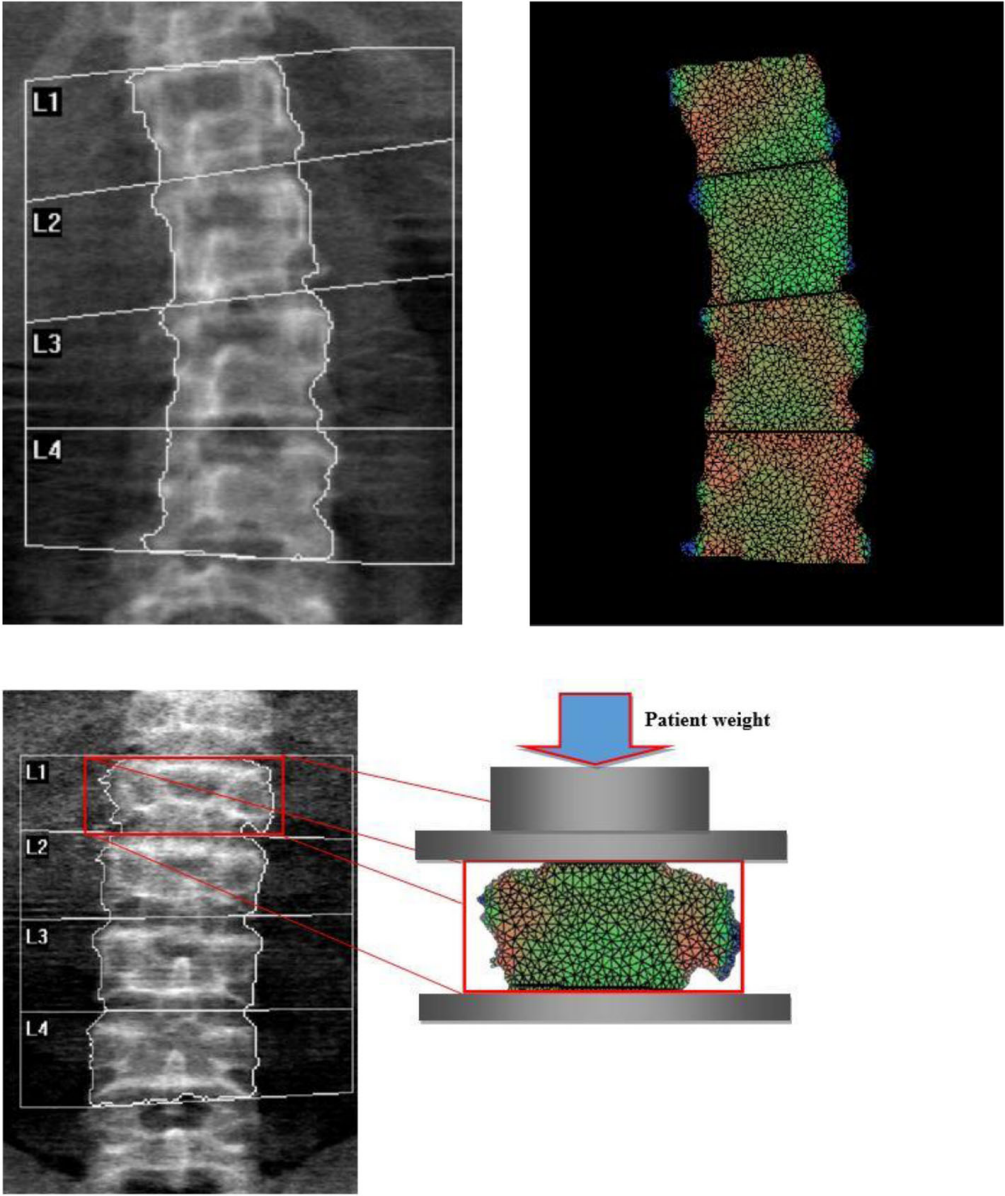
Example of a lumbar dual-energy x-ray absorptiometry (DXA) scan in the absence of fracture (upper left) and its bone strain index distribution (upper right). Example of a spine DXA scan (bottom left) and of bone strain index distribution for a fractured lumbar vertebra (bottom right) after distribution
